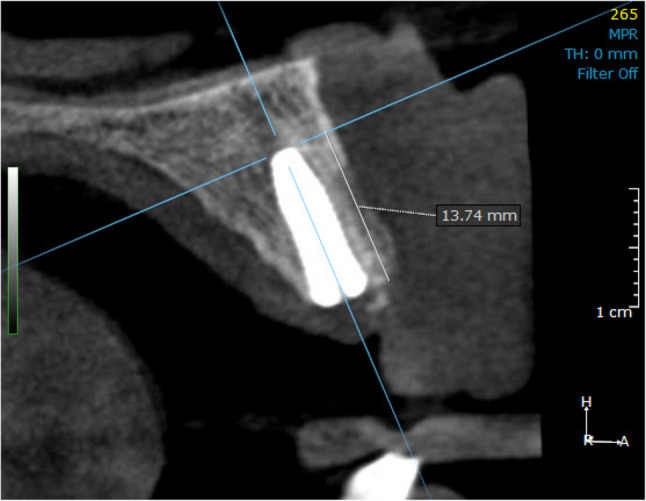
Measurement of MBL. A cross sectional CBCT image showing measurement of MBL represented by white line parallel to the implant, and its height was measured in millimeters at the crest of the buccal plate of bone and ending at the apical level of the implant. The implant was utilized as a reference for the measurement of MBL from the cross-sectional view by adjusting panoramic long axis in its center and bisecting it (showing the buccolingual dimensions) represented by blue lines
